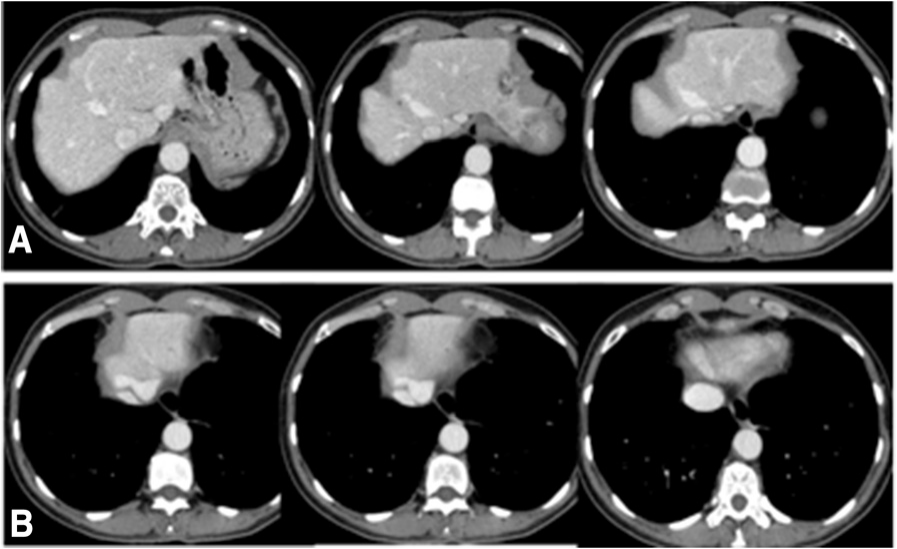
Abdominal CT with dynamic study in the portal phase in axial plane on case 2 (Type 2). Note: a: axial plane on the topography of venous ligament, highlighting the vascular route along the caudate segment and converging path of hepatic veins to the inferior vena cava. Featured of the extrahepatic portal vein hinting at the venous ligament. b: In the most cranial axial liver, showing the junction of the extrahepatic portal vein with the medial aspect of the inferior vena cava
